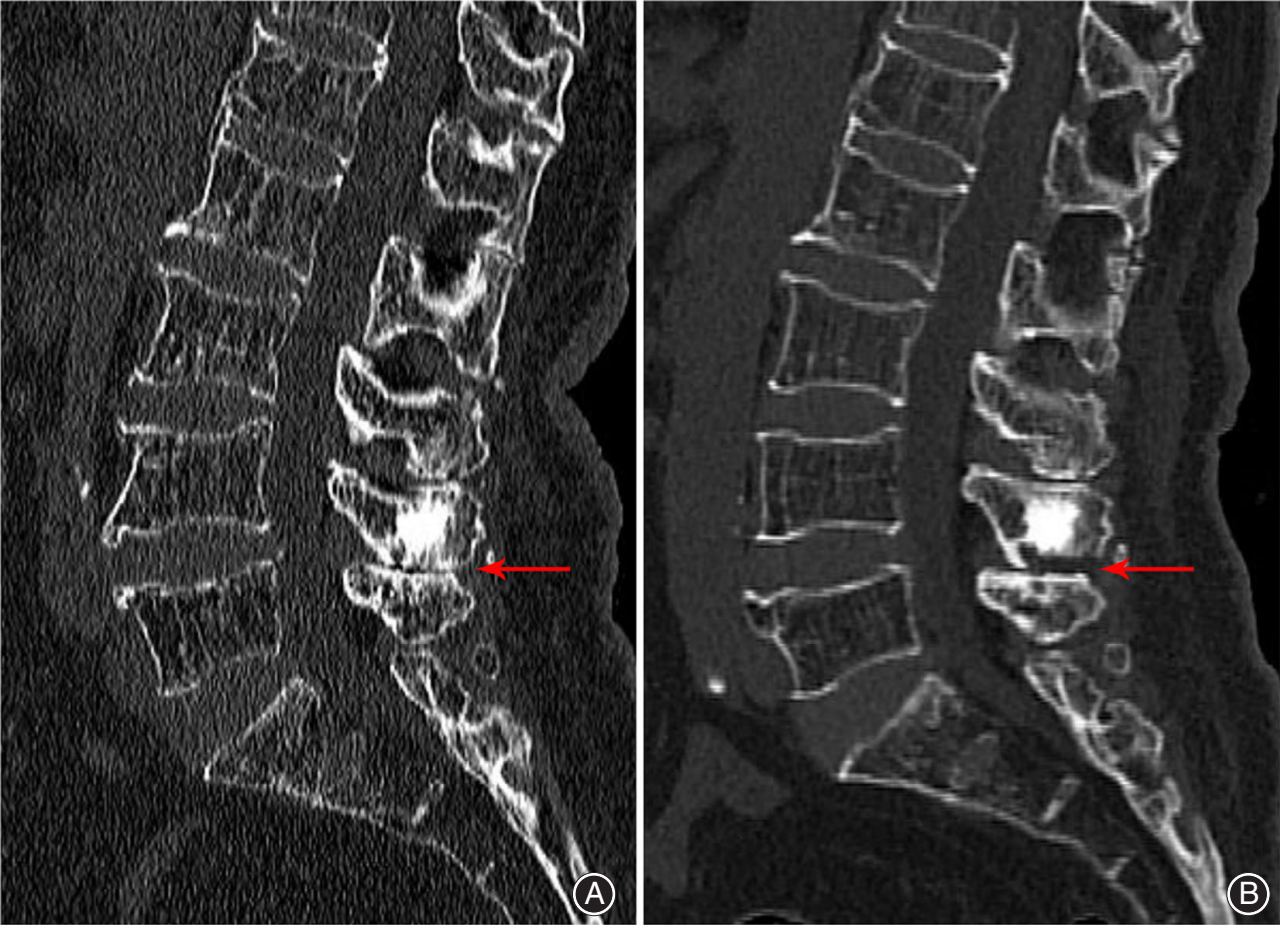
Compared with the preoperative CT images (A), the postoperative CT (B) showed that the distance between the L_4_ and L_5_ spinous processes had been enlarged from 1 mm to 4 mm.
